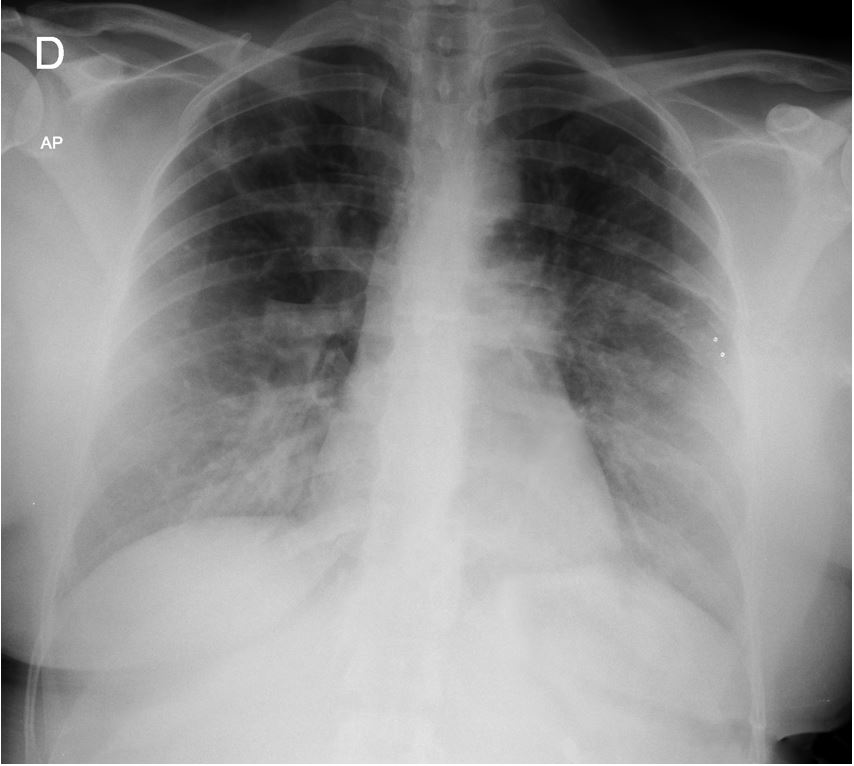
An anteroposterior chest radiograph at admission demonstrating bilateral interstitial infiltrates.
